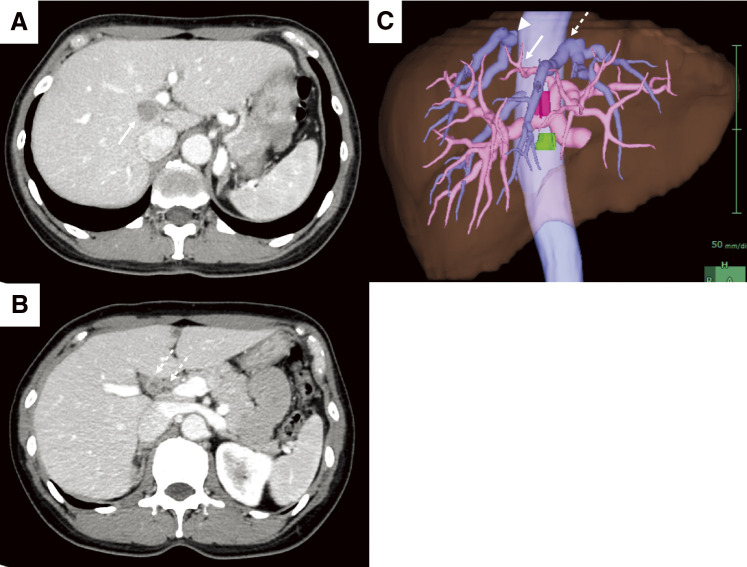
Enhanced CT and 3D reconstruction images before chemotherapy. (A) A 19 mm low-density tumor (arrow) is seen at the origin of the right and left branches of the portal vein and the abnormal P7. (B) A 12 mm contrast-enhanced lymph node (dotted arrow) is observed in the hepatoduodenal ligament. (C) An abnormal portal branch of segment 7 (arrow) branches from the umbilical portion of the portal vein and runs dorsal to the middle hepatic vein (dotted arrow) and the right hepatic vein (arrowhead). The portal vein is shown in pink, and the hepatic vein is shown in light blue. Tumors are shown in red and lymph node metastases are shown in green. CT, computed tomography
